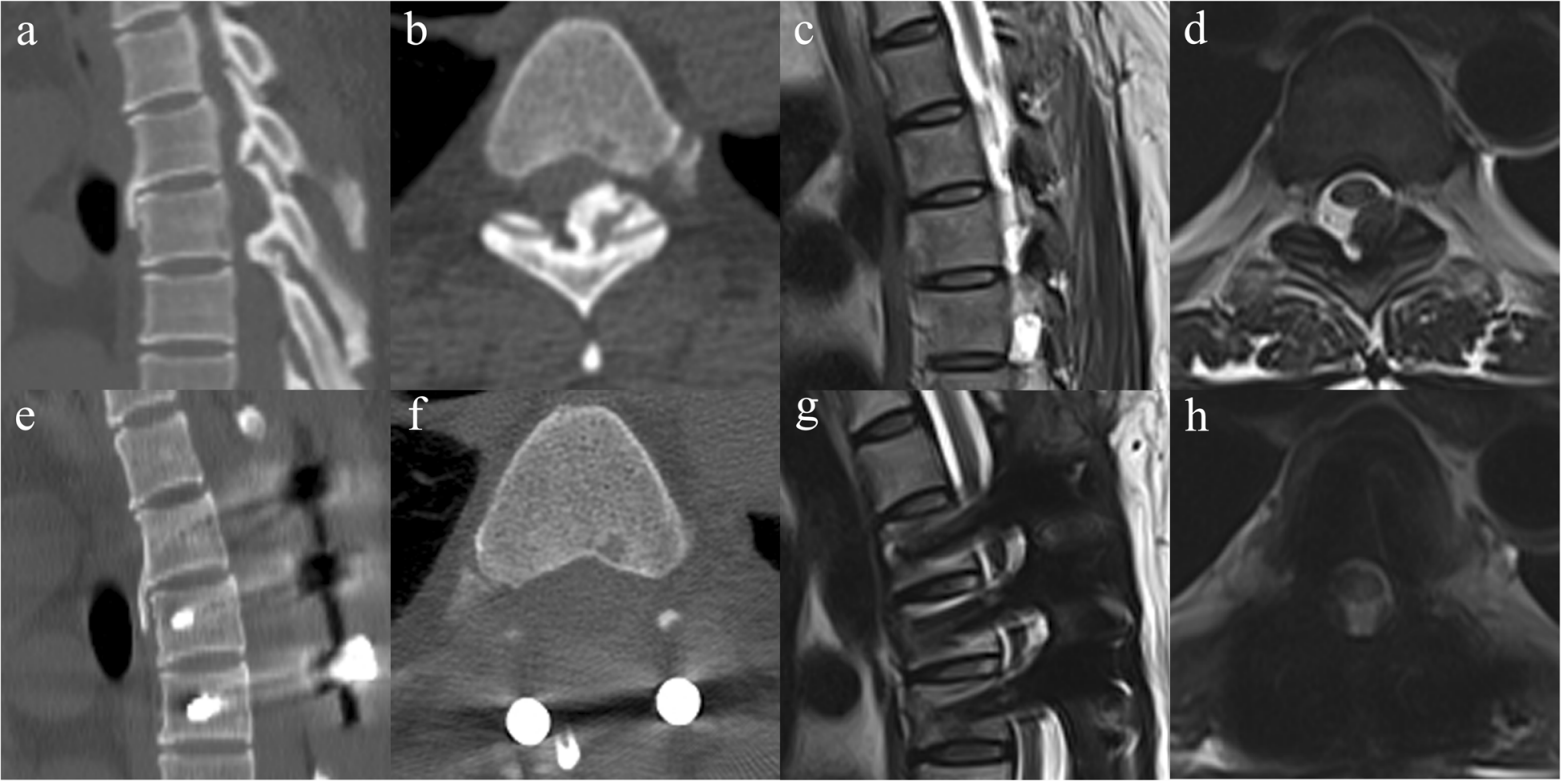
A patient with OLF received intraoperative MP. Preoperative CT and MRI show OLF at T3-T5 (a-d). The larger ossified ligament flavum (T4-T5) is unilateral (b, axial CT) and round type (c, sagittal MRI), with no T2 high intensity at corresponding spinal cord on MRI (d, axial MRI). Postoperative CT and MRI demonstrate good decompression (e-h). One week after surgery, wound infection was found. Bacteria culture result was methicillin-sensitive *Staphylococcus aureus*. Treatment methods were intravenous vancomycin and local wound care. The patient then made a full recovery without further infection symptoms
